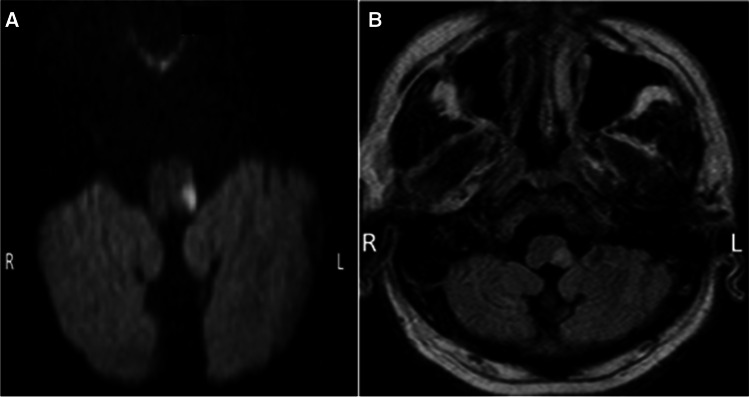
MRI brain, A axial DWI, and B FLAIR show an acute left-sided dorsal lateral medullary infarct
